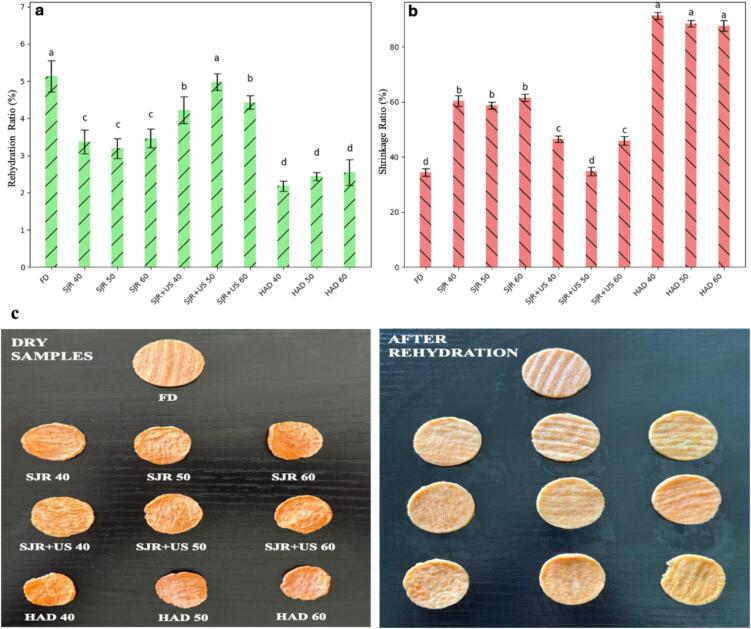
A) rehydration ratios of dried sweet potato samples, b) shrinkage ratios of dried sweet potato samples, c) effect of drying methods and rehydration on physical appearance of samples. *Note:* FD refers to freeze-dried sweet potatoes; SJR 40, SJR 50, and SJR 60 refer to sweet potatoes dried with slot jet reattachment (SJR) nozzle at 40 °C, 50 °C, and 60 °C, respectively; SJR + US 40, SJR + US 50, and SJR + US 60 represent sweet potatoes dried with ultrasound (US) and slot jet reattachment (SJR) nozzle at 40 °C, 50 °C, and 60 °C, respectively; HAD 40, HAD 50, and HAD 60 correspond to sweet potatoes dried with hot air drying (HAD) at 40 °C, 50 °C, and 60 °C, respectively.
